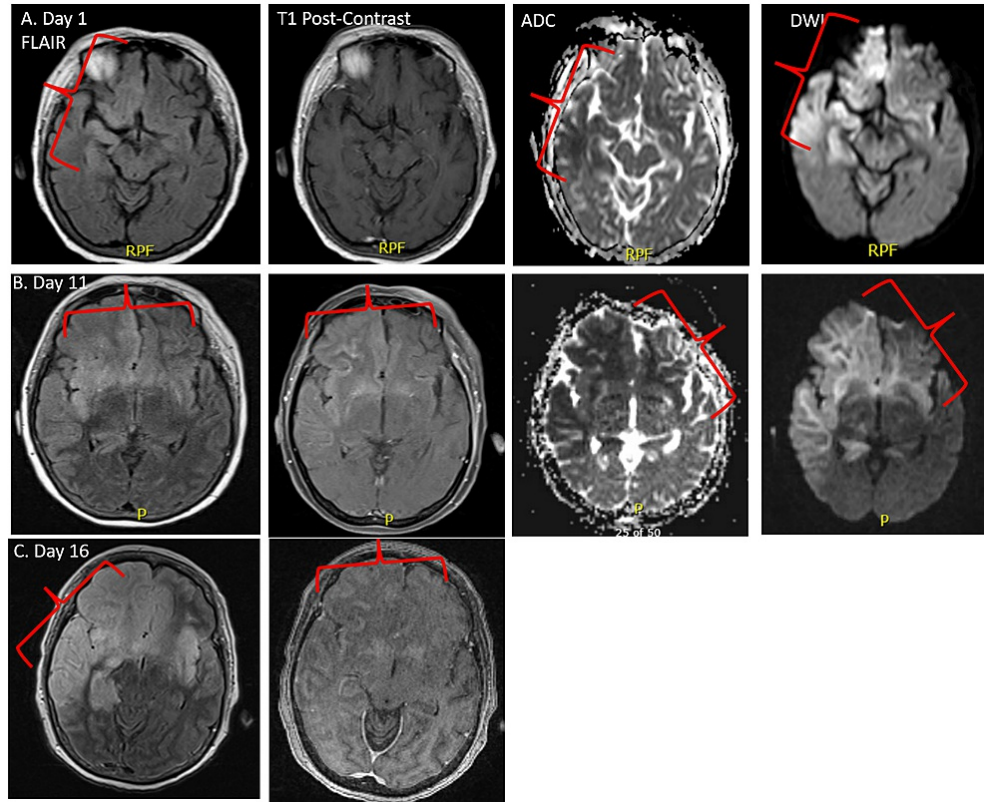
Radiologic progression of disease seen on MRI brain A) Day 1: MRI brain FLAIR demonstrates right temporal and frontal lobe areas of hyperintensity and ADC/DWI mismatch consistent with HSV encephalitis and acute infarction (red arrows). B) Day 11: MRI brain FLAIR shows progression to bilateral involvement. C) Day 16: MRI brain shows severe bilateral frontotemporal enhancement. FLAIR: fluid-attenuated inversion recovery; ADC: apparent diffusion coefficient; DWI: diffusion-weighted imaging; HSV: herpes simplex virus
